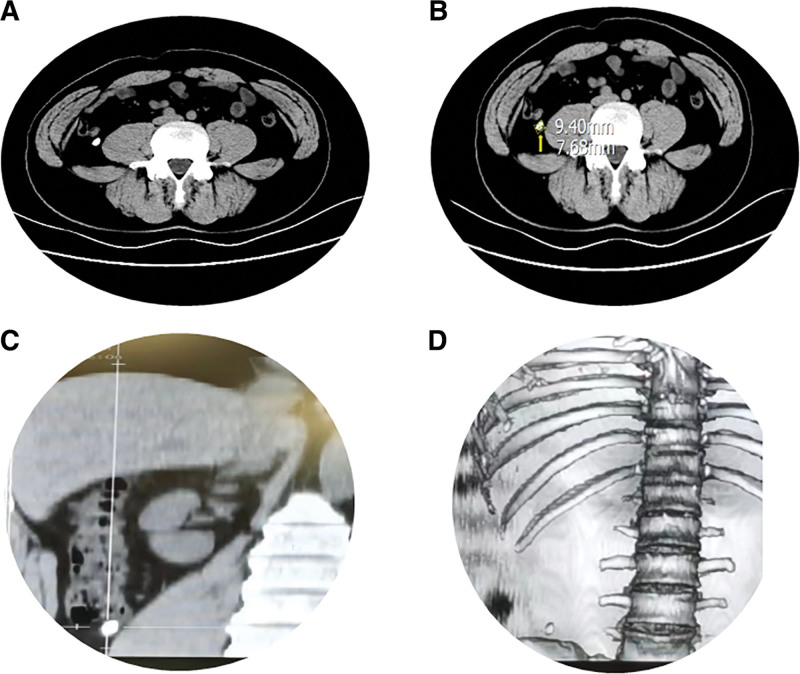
Abdominal CT scan before treatment. (A) Axial non‐contrast CT image shows a nodular hyperdense lesion in the appendiceal region of the right lower quadrant, consistent with appendicolith. (B) Axial non‐contrast CT image with linear measurements of the appendicolith. (C) Coronal reconstructed CT image clearly depicts the distal course of the appendix and the location of the appendicolith. (D) Three-dimensional volume‐rendered CT image shows the density comparison between the appendicolith and adjacent bone, suggesting the calcified characteristic of the stone. CT = computed tomography.
